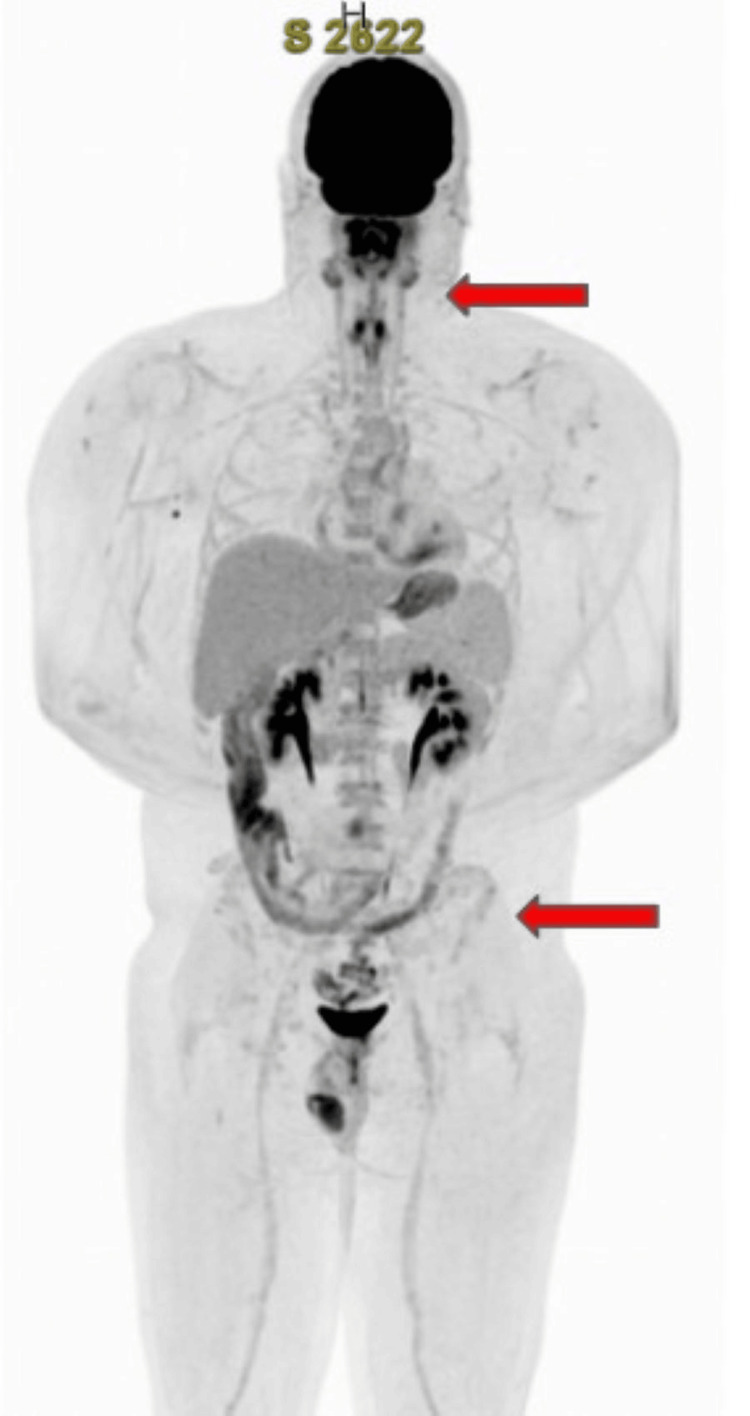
PET/CT scan after two autologous stem cell transplants showed similar lytic bone lesions without abnormal increased FDG uptake. PET/CT= Positron emission tomography/computed tomography scan, FDG=fluorodeoxyglucose
